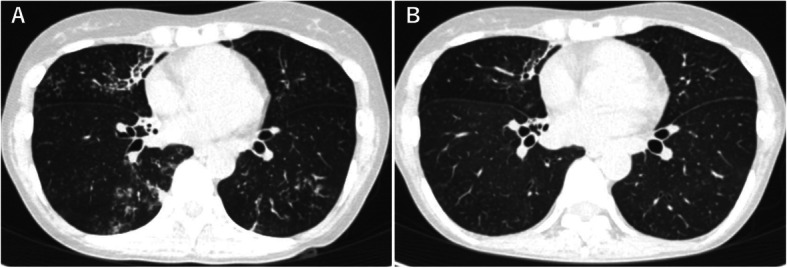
Chest CT before treatment shows small centrilobular nodules in the middle and lower lobes and slight bronchiectasis with a consolidation in the middle lobe (a). After 2 months treatment, small centrilobular nodules almost vanished (b)
